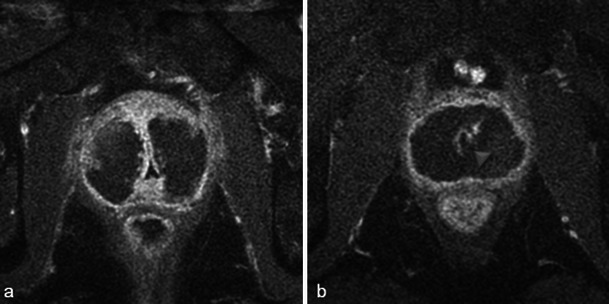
Periurethral enhancement ring. Day-7 post-treatment axial T1 FS contrast-enhanced MR images for a bilaterally treated patient show necrosis in both lobes. The intraprostatic urethra presents a continuous enhancement ring (a). A rupture of this ring enhancement (*arrowhead*) after photodynamic therapy is considered suspicious of urethral wall necrosis (b). No urinary complications were identified in this patient during close clinical follow-up
